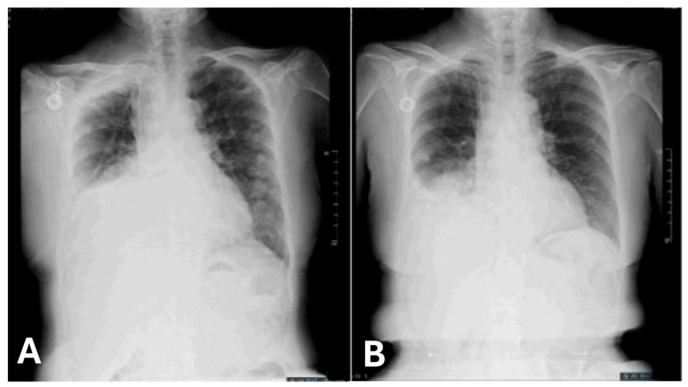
Timeline of the patient’s chest x-ray, showing a right-sided massive pleural effusion and an excellent response to continuous treatment: (A) 12 January 2024, and (B) 4 March 2024.
